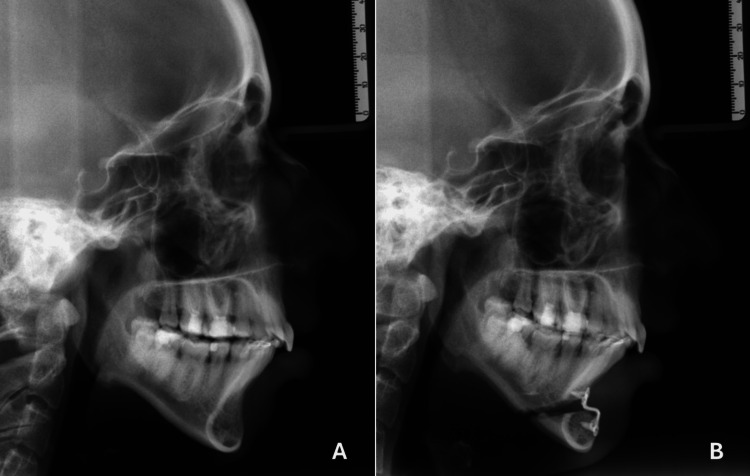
Genioplasty (radiographic view) A) Pre-operative radiographic study and B) post-operative radiographic study Part of the personal archive of the authors
